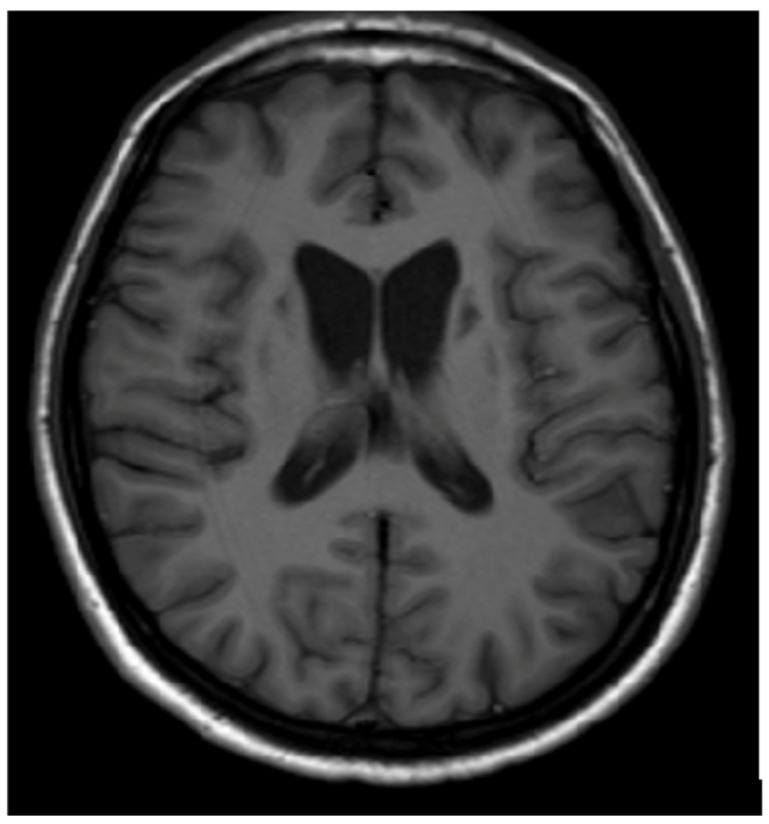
Hypointense changes localized in both putamen in T1-weighted images (own materials of the neurology department).
